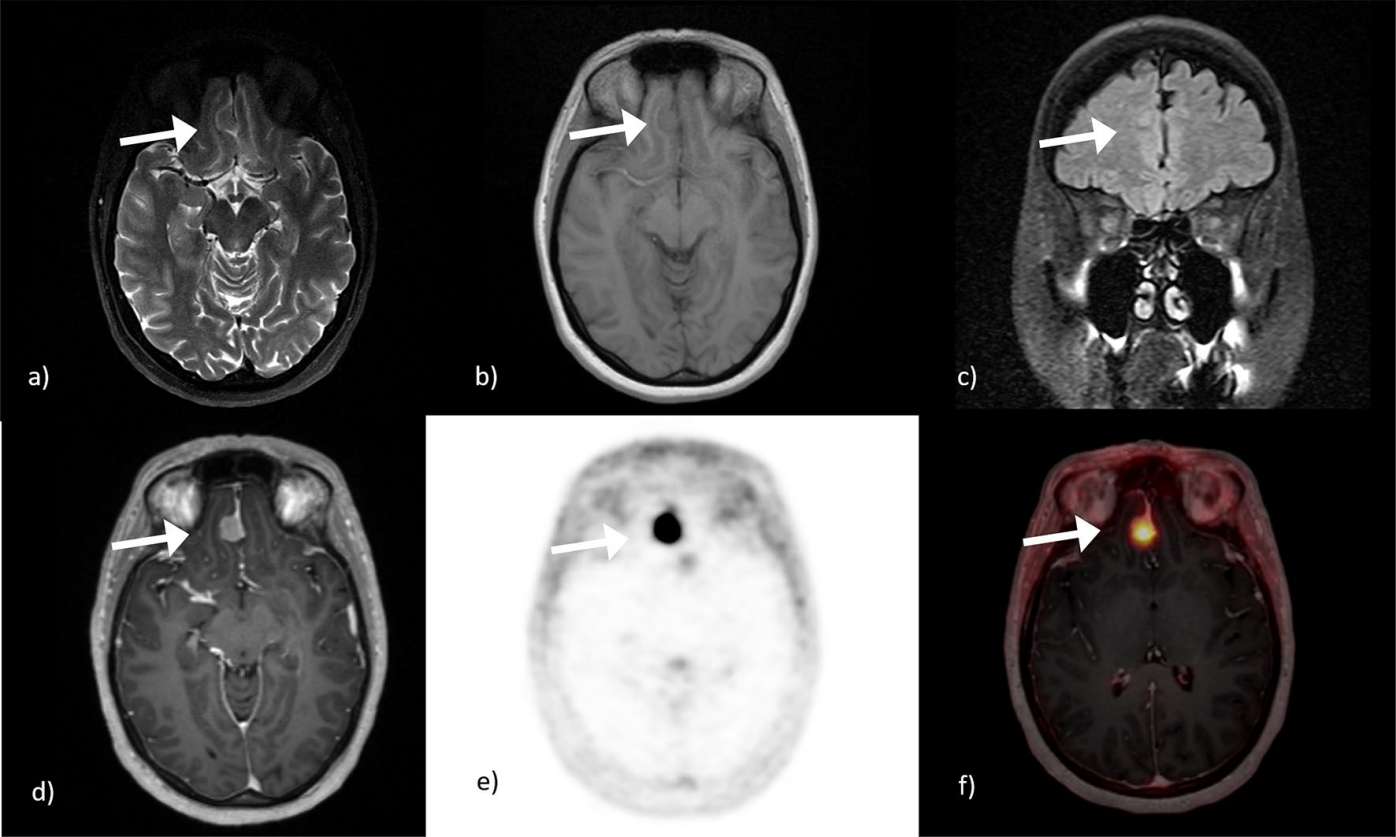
61 years old female patient with a typical falcine meningioma in the front. The first row presents the difficulty in differentiation the meningioma from the surrounding parenchyma due to isointensity. Here presented for (a) T2 TSE with CSF cleft sign, (b) T1 FL2D and (c) TIRM without edema. However the falcine meningioma can be easily detected after contrast medium injection in MPRage (d) after 68 Ga-DOTATOC injection for PET (e) and in fusioned imaging PET/MRI (f).
